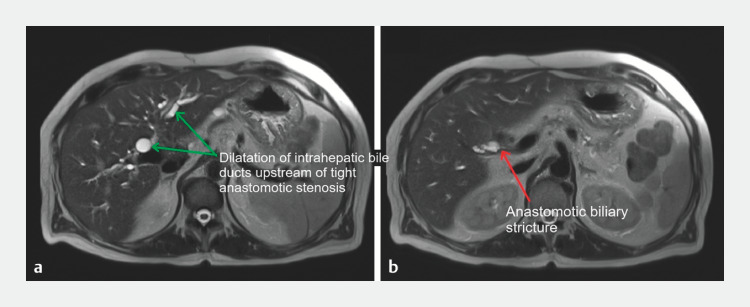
Cross-sectional magnetic resonance imaging (MRI) image showing a disparity in caliber between the recipient bile duct and the graft bile ducts.
